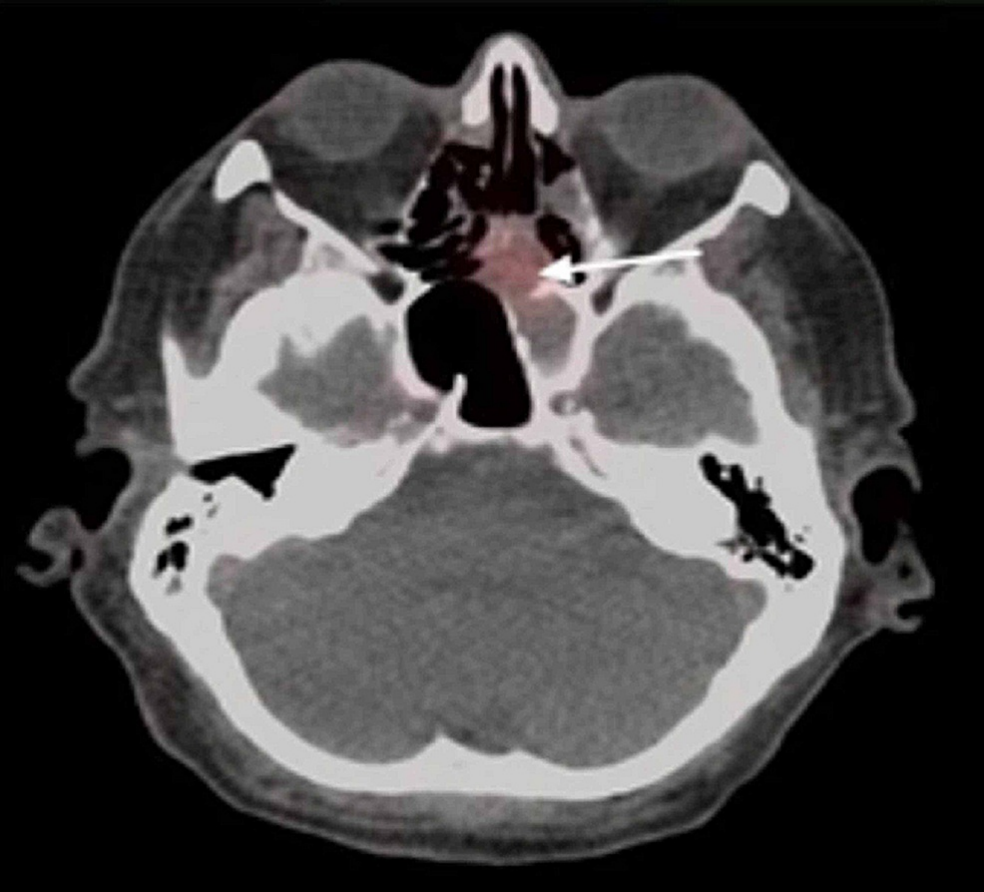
FDG PET CT shows increased uptake in the soft tissue structure that extends from the sphenoid sinus into the ethmoid sinus which can be secondary to neoplastic or inflammatory process. But since this patient has pathologically enlarged lymph nodes, a neoplastic process cannot be excluded. Tissue sampling was indicated for further evaluation. FDG: fludeoxyglucose, PET: positron emission tomography, CT: computed tomography
